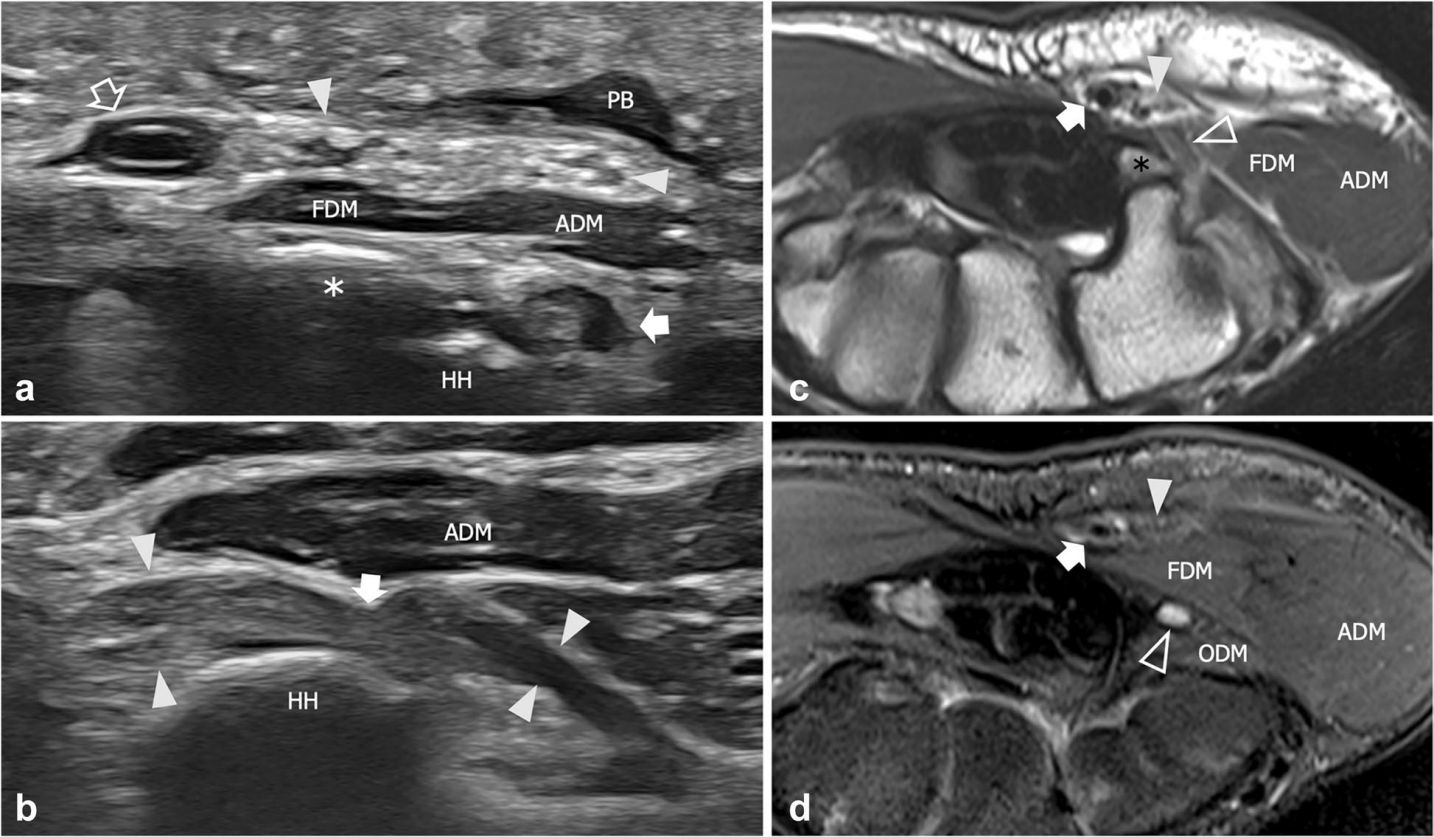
Deep branch impingement against an unrecognized hamate hook fracture in a 40-year-old male with motor impairment in the territory of the ulnar nerve after a blunt trauma over the palm. a Short-axis 22–8-MHz US image demonstrates severe edematous changes and swelling of the deep branch (arrow) as it runs on the ulnar side of the hamate hook (HH). A small piece of fractured bone (asterisk) is shown avulsed from the tip of the hook. The terminal divisions of the superficial branch (arrowheads) present a normal appearance as they run in between the palmaris brevis (PM) and the abductor digiti minimi (ADM) and flexor digiti minimi (FDM). Outlined arrow, ulnar artery. b Long axis 22-8MHz US image shows a swollen deep branch (arrowheads) presenting a distorted path around the level of the hamate hook. Note the abrupt change in caliper of the nerve at the hiatus (arrow), related to the impingement. c Transverse turbo Spin-Echo T1-weighted and d transverse turbo Spin-Echo fat suppressed T2-weighted MRI scans confirm swelling and edematous changes of the deep branch (outlined arrowhead) and the presence of a fracture of the hamate hook (asterisk). The ulnar artery (arrow) and the superficial branch (arrowhead) have a normal appearance
